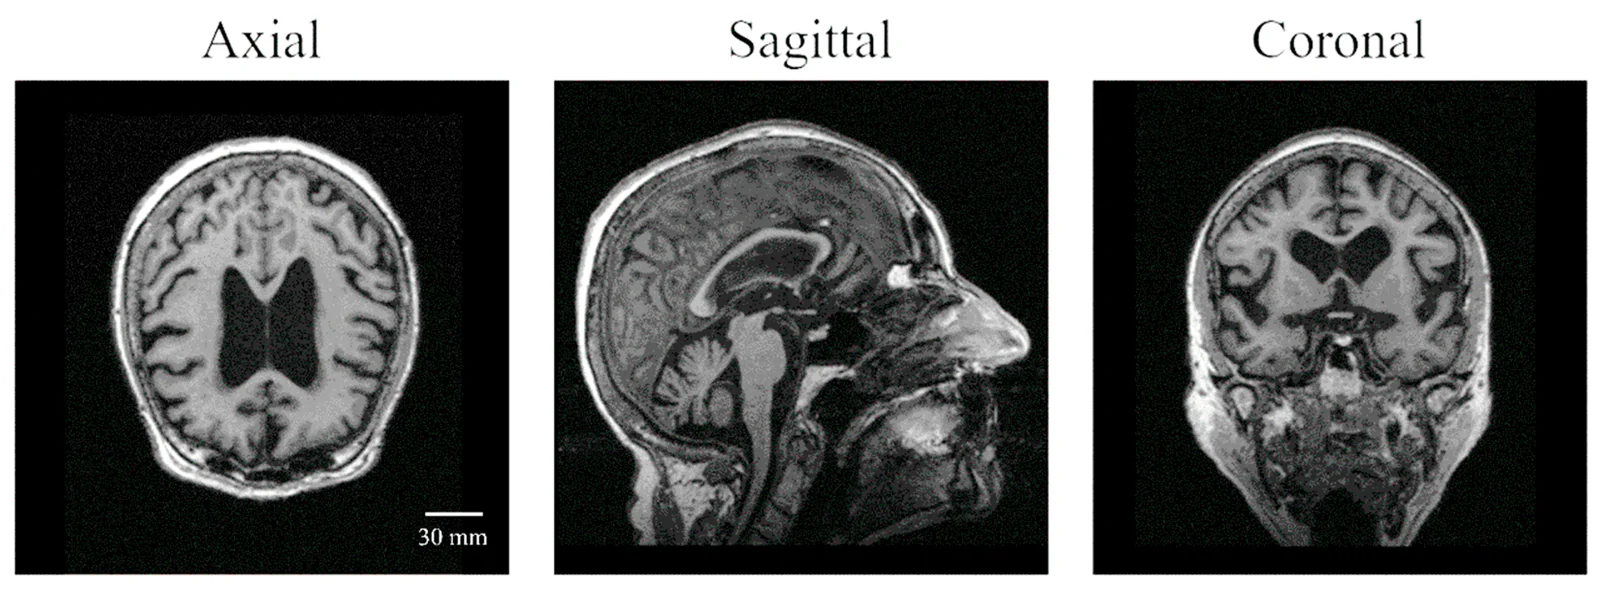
Representative axial, sagittal, and coronal T1-weighted images from ADNI.
